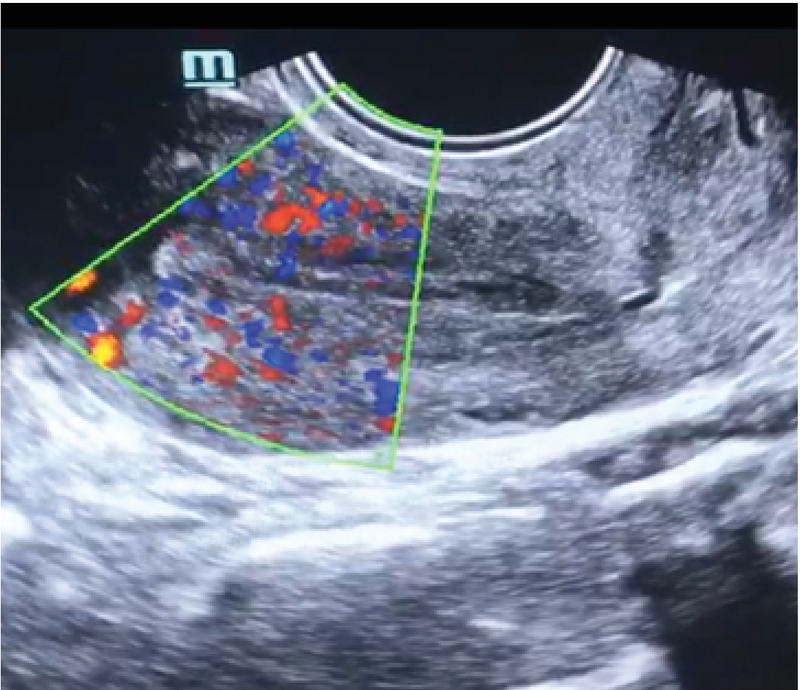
Endometrial vascularity in 2D ultrasound with Color Doppler.
